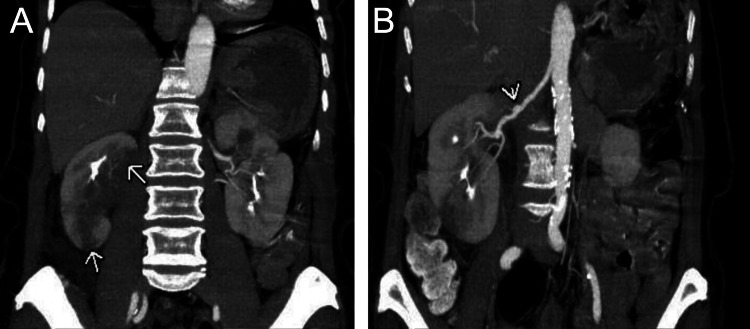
Coronal CTA A: Large wedge-shaped regions of hypoperfusion in the upper pole and lower pole of the right kidney with sharp demarcated margins (arrows). Differential includes renal infarcts, pyelonephritis, and neoplasm. B: Given the beaded appearance of the right renal artery, renal infarct from FMD is likely (arrow). CTA: computed tomography angiography
